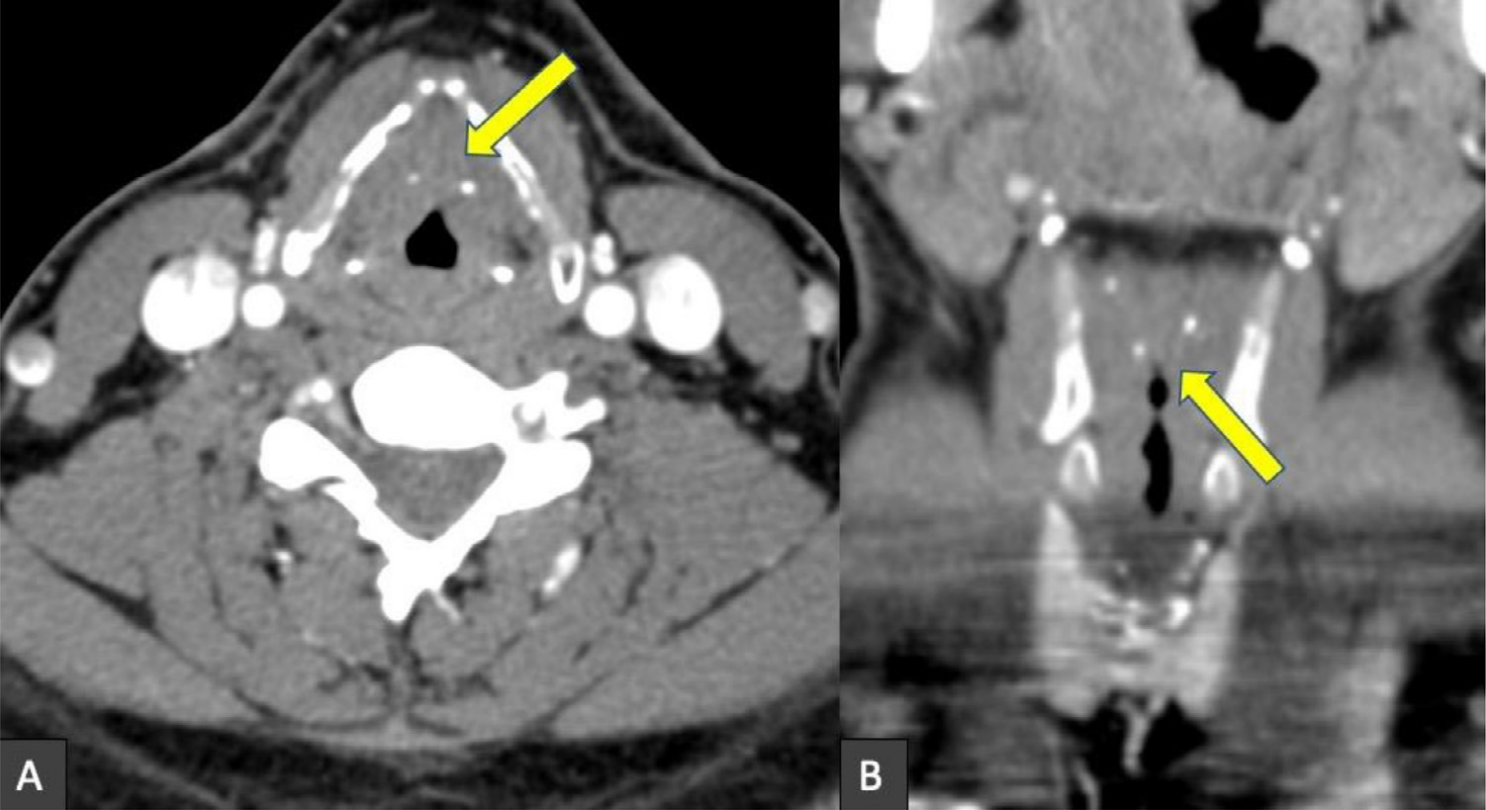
Axial (A) and coronal (B) contrast-enhanced CT of the neck demonstrates nodular thickening of the vocal folds with few coarse calcifications. There is associated airway narrowing, likely resulting in the dysphonia encountered in our patient.
